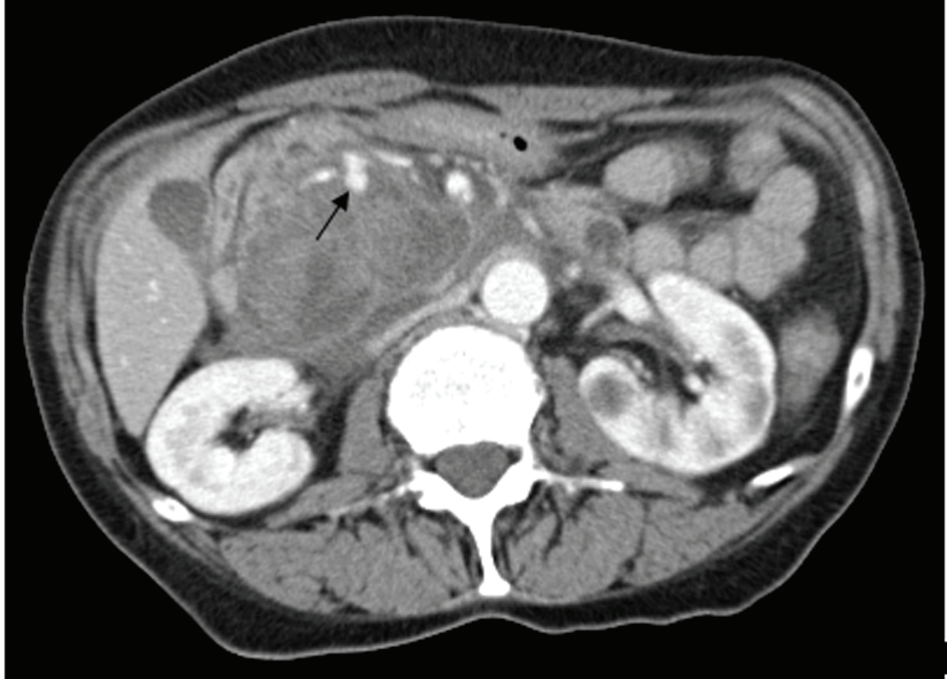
Contrast-enhanced CT scan of the abdomen reveals a 5 × 6 × 7 cm complex cystic mass in the region of uncinate process of pancreatic head with an enhancing capsule and a small hyperdensity consistent with pseudoaneurysm of a peripancreatic vessel with active bleeding into the pancreatic pseudocyst.
